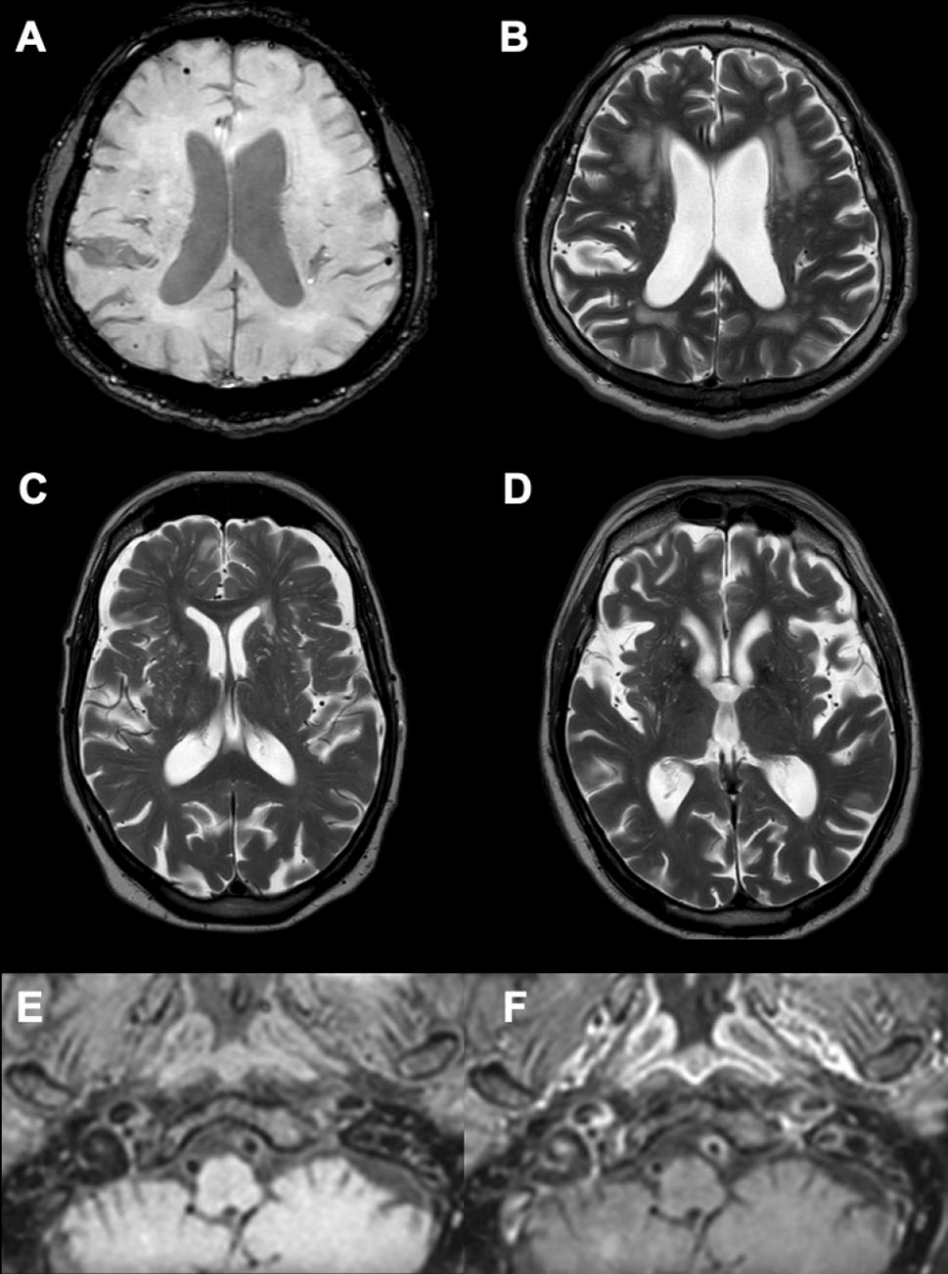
Imaging features explored: microbleeds on SWI (A), white matter hyperintensities (B), EPVS (C), and lacunes (D) on T2-weighted, and endotheliitis as suggested by enhancement of the vessel wall of the left vertebral artery on VISTA sequences after gadolinium injection (F vs pre-contrast E)
